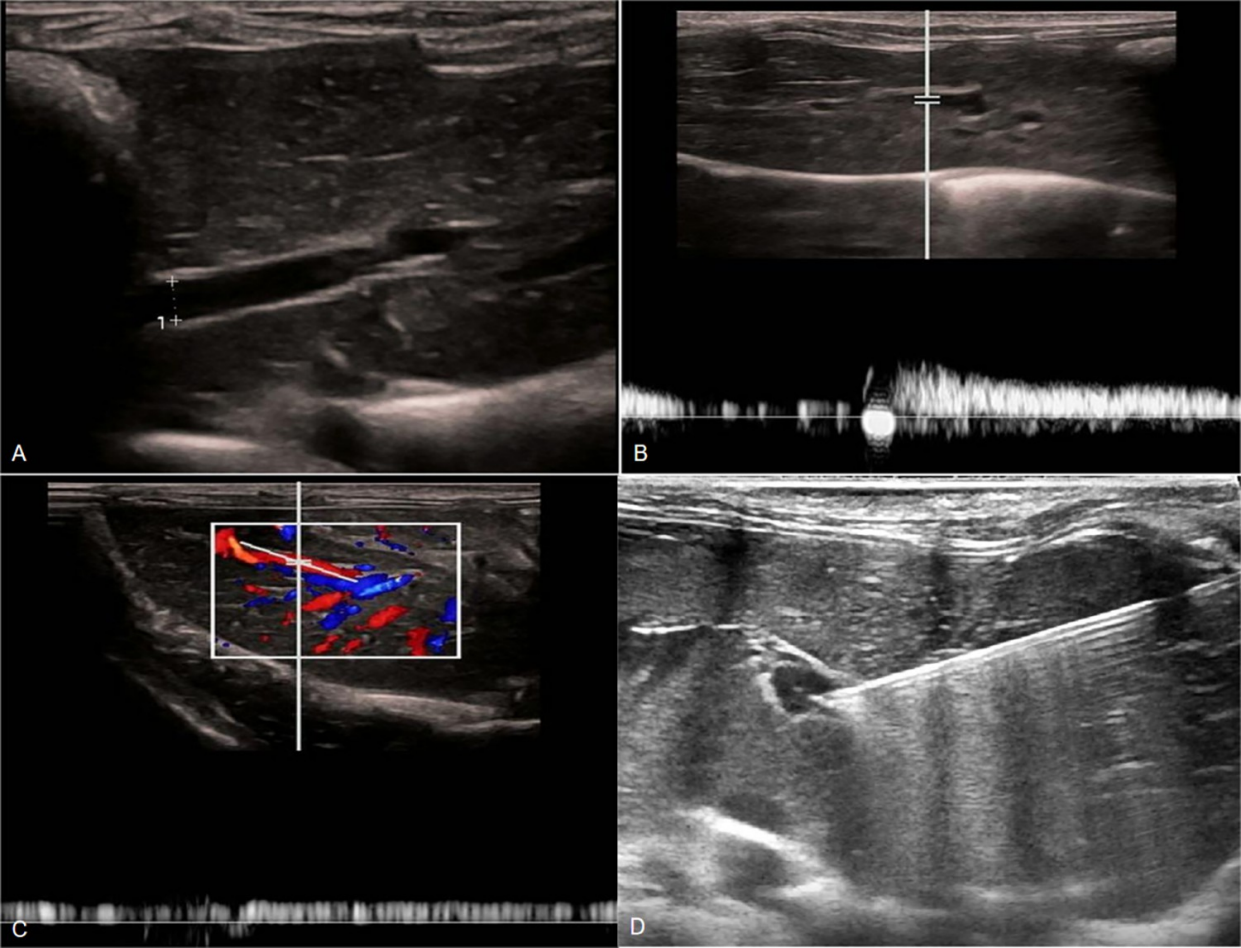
Measurement of inner diameter, flow rate, and pressure of portal vein under ultrasound guidance. A: Measurement of inner diameter of portal vein; B, C: Measurement of flow rate of portal vein; D: Measurement of PVP by puncture.
